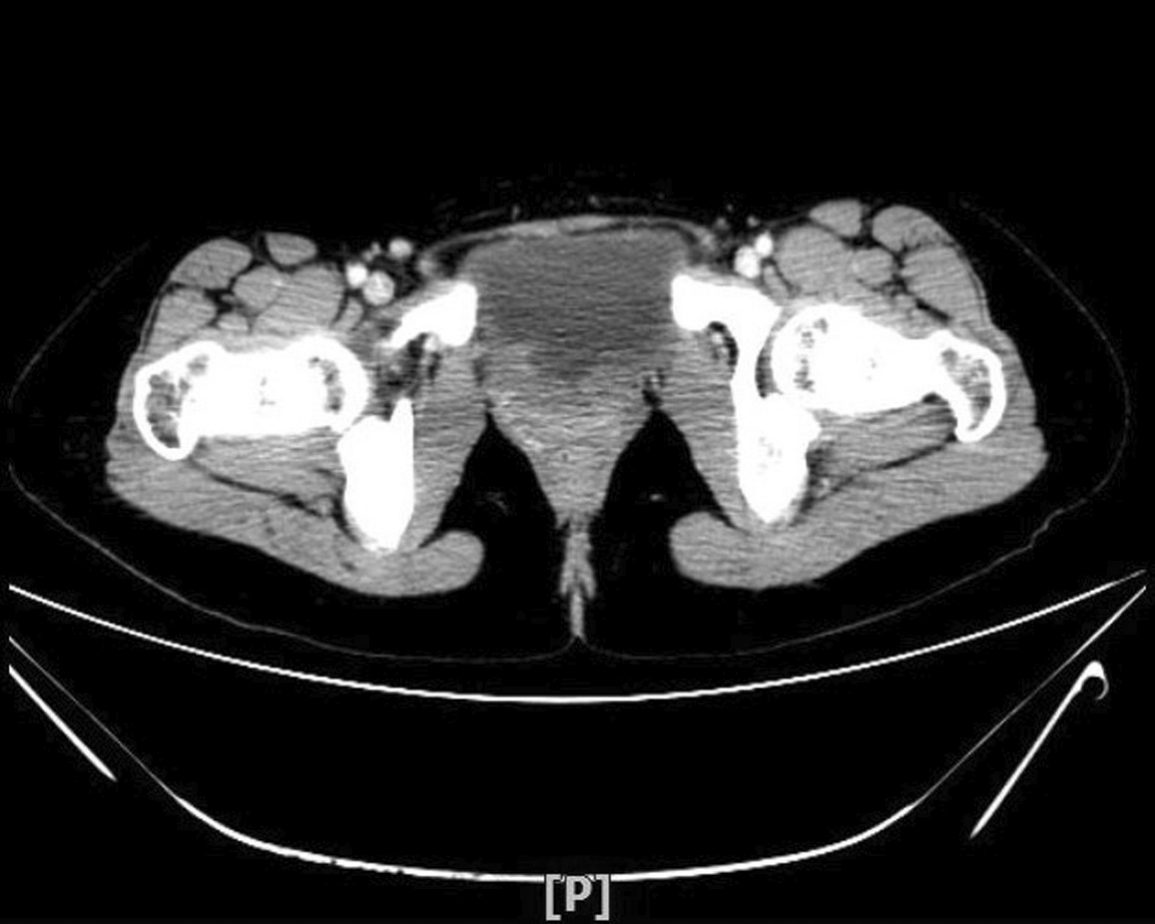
In the CT scan, a small polypoid lesion in the right side of the bladder base (5 mm) with no perivesical infiltration.
